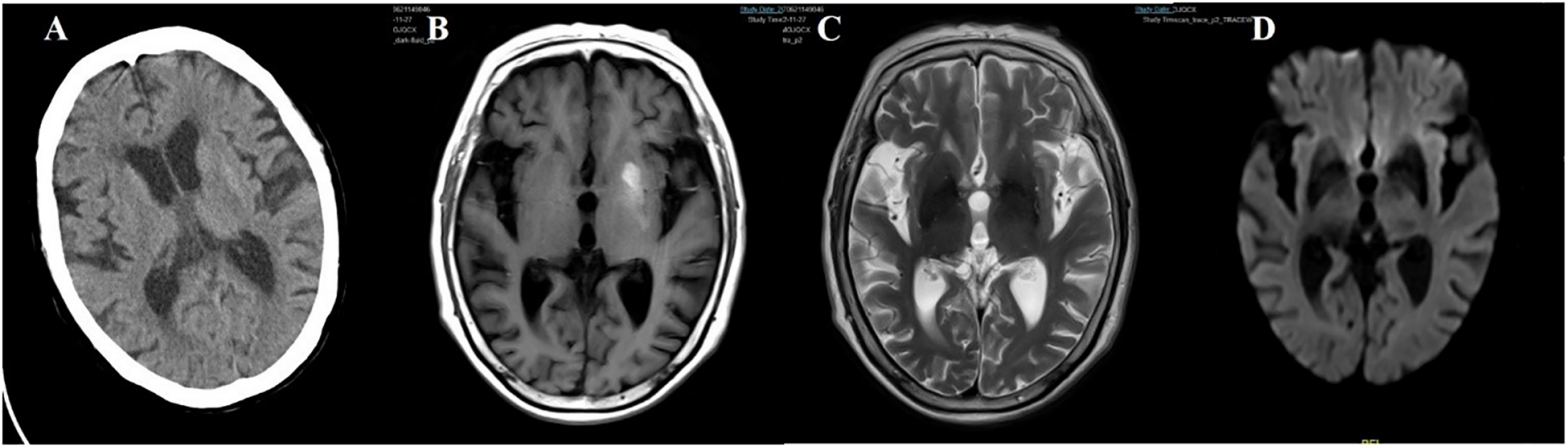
Case 8. (A) Brain CT scan demonstrated a slightly higher density in the left putamen. (B) Brain MR T1 image demonstrated hyperintensity in the left putamen. (C) Brain MR T2 image revealed hypointensity in the bilateral putamen. (D) The DWI sequence showed low signals in the bilateral basal ganglia.
